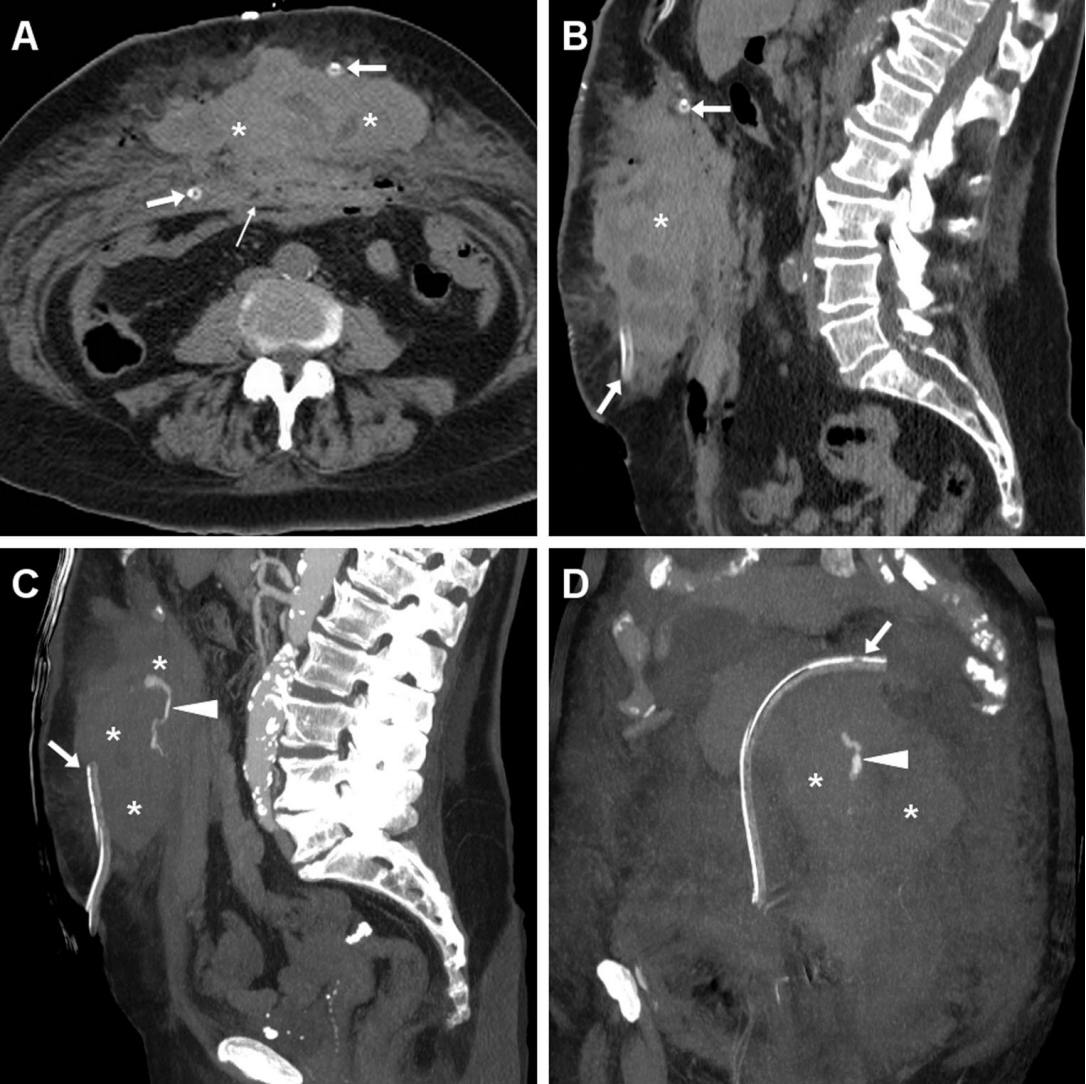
Active post-surgical bleeding in an elderly 84-year-old female with acute abdominal pain, hard-consistency swelling, and severe blood loss (8 g/dl nadir haemoglobin) 24 hours after Rives-Stoppa repair of a midline incisional hernia. Emergency CT depicted a 15x6x13 cm hyperattenuating (median 55 HU) fresh haematoma (*), extending ventrally from the surgical site through the rectus muscles and subcutaneous fat. Note PM (thin arrows) and drainage tubes (arrows). Sagittal (c) and coronal (d) MIP reconstructions showed serpiginous contrast extravasation (arrowheads) isoattenuating with the enhanced aortic lumen within the haematoma, consistent with active arterial bleeding. Relaparotomy confirmed bleeding suprafascial haematoma, which required haemostasis and surgical drainage [Partly reproduced with permission from Ref. no. [18]]
